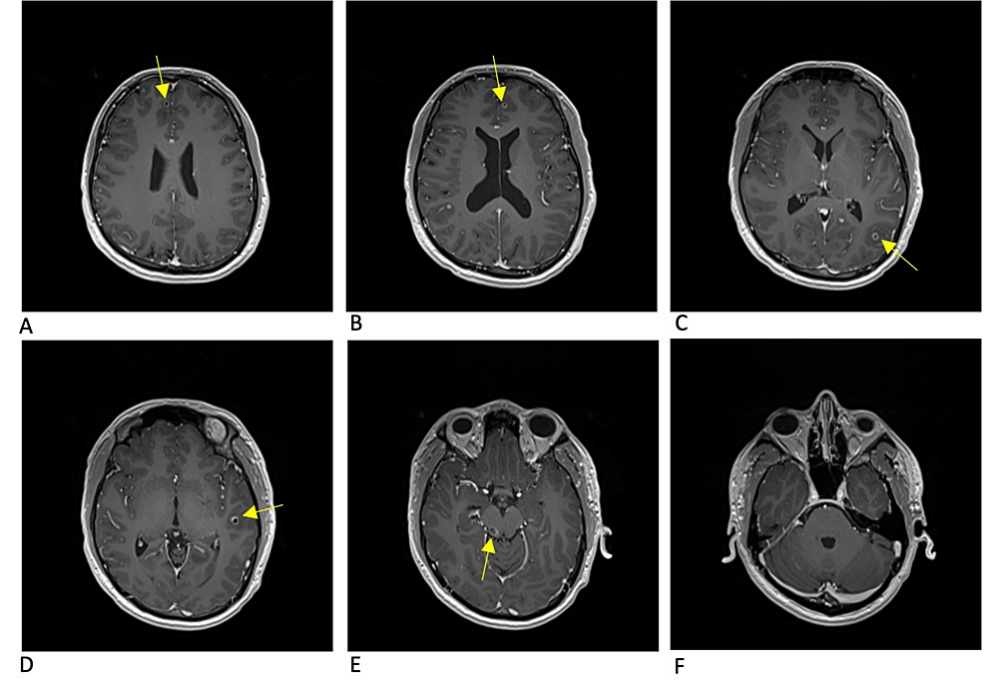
MRI brain T1 axial post-contrast sequence at the onset of symptoms There are multiple bilateral ring-enhancing lesions as denoted by arrows. Multiple cystic lesions can be found in the anterior right and left frontal lobes (panes A and B). Cystic lesions with increased amounts of perilesional edema can be found in the posterior left parietal lobe and left temporal lobe (panes C and D). The lesion located in the right posterior midbrain is the largest of all cysts with a significant amount of perilesional edema (pane E). Lesions depicted are in the colloidal to granular-nodular stages. Note: Pane F demonstrates the absence of cysts in the cerebellum.
